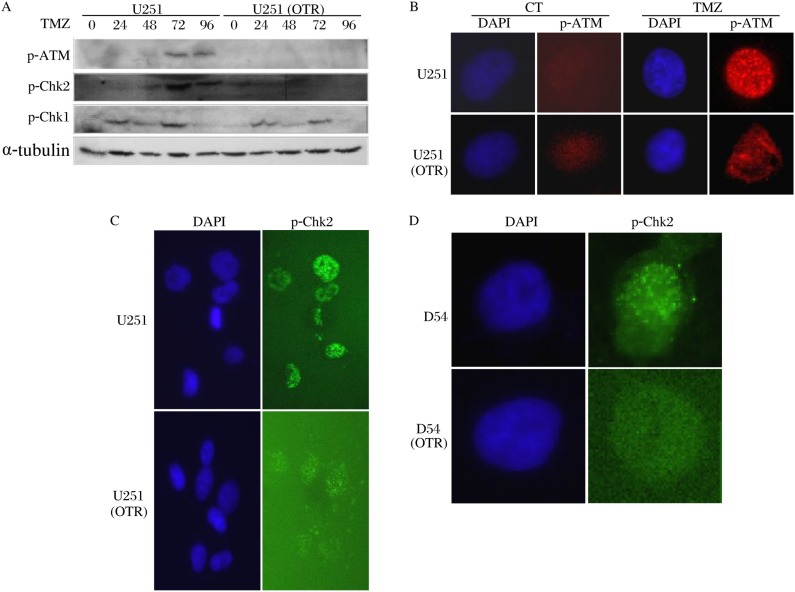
The resistant GBM cells exhibit defective signaling through the ATM-Chk2 pathway. A: U251 (OTR) fails to activate the ATM-Chk2 pathway in response to TMZ. The Western blotting were probed with phospho-specific antibodies against ATM (p-ATM), Chk1 (p-Chk1) and Chk2 (p-Chk2). B: TMZ induces focus formation by p-ATM in U251, but not in U251 (OTR). C and D, TMZ induces focus formation by p-Chk2 in D54 and U251, but not in their TMZ-resistant counterparts. The cells in B, C and D were analyzed at 72 h post TMZ treatment. CT: control; TMZ: temozolomide.
